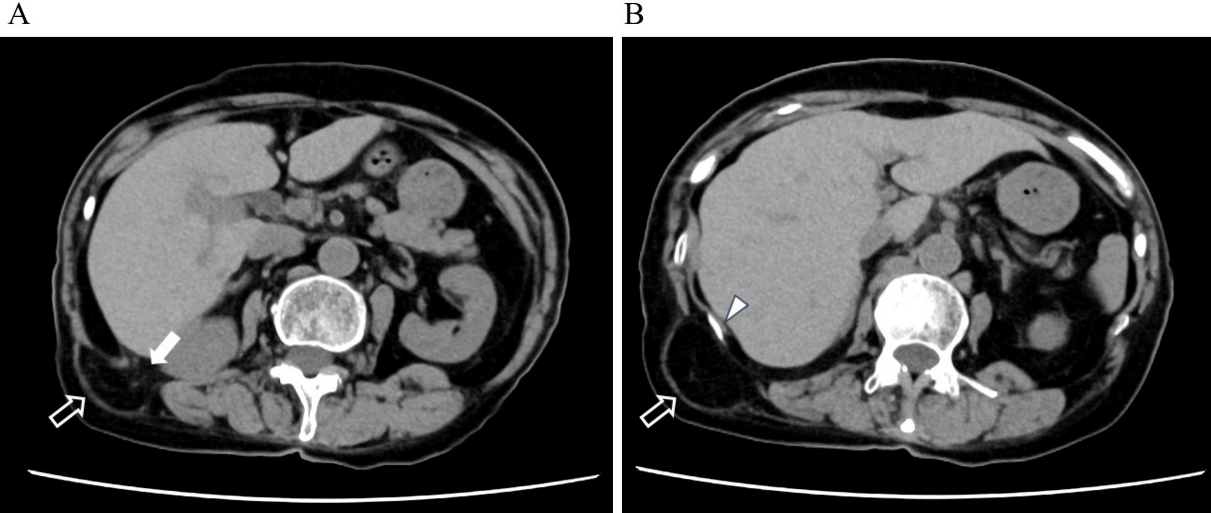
Axial CT images demonstrating a right superior lumbar hernia. (A) Retroperitoneal fat (hollow white arrow) is seen protruding through the hernial orifice (solid white arrow), located posterior to the right kidney and lateral to the liver. The fat extends into the subcutaneous tissue through the presumed location of the superior lumbar triangle (Grynfeltt’s triangle). (B) A more cranial slice than (A), with the arrow indicating the 12th rib. The continuity of the fat protrusion in an upward direction is clearly observed, with no involvement of bowel or other intra-abdominal organs. CT: computed tomography.
